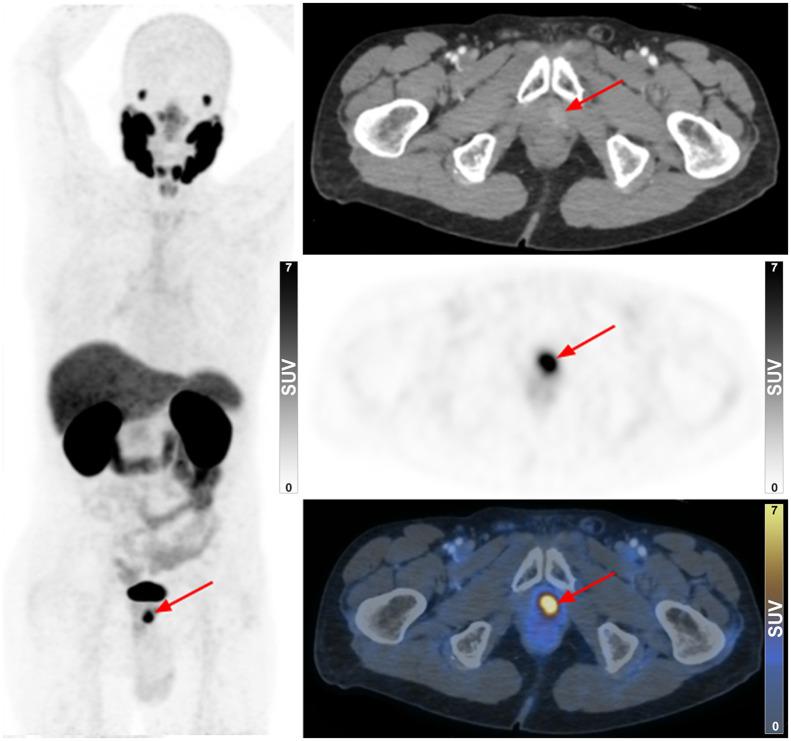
^68^Ga-PSMA-11 PET/CT of 84-y-old B(−) man with rising PSA levels. Focus of increased uptake was identified at left apex of prostate (arrow), indicating localized disease.
